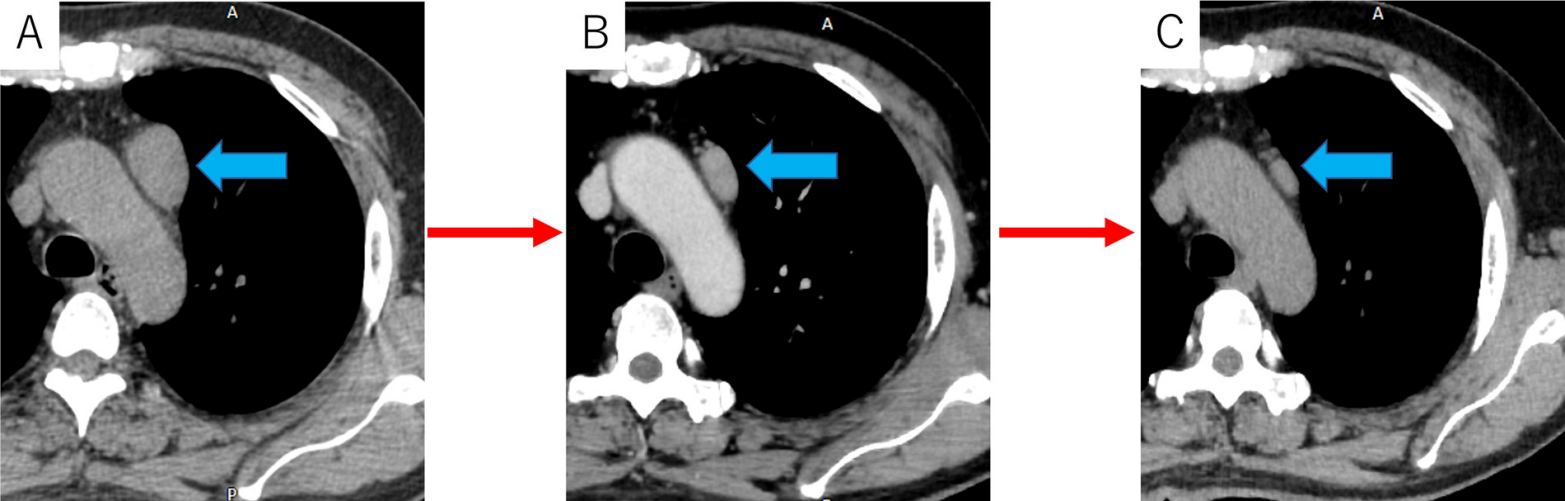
(A) The anterior mediastinum mass was smooth and 45 mm in maximum diameter on nonenhanced CT acquired at the hospital previous to the patient's consultation at our hospital (arrow). (B) One month later, contrast-enhanced CT of the chest during the patient's first visit to our hospital showed that the mass had regressed to 31 mm (arrow). (C) Another month later, a chest CT examination before surgery revealed further tumor regression to 27 mm (arrow).
